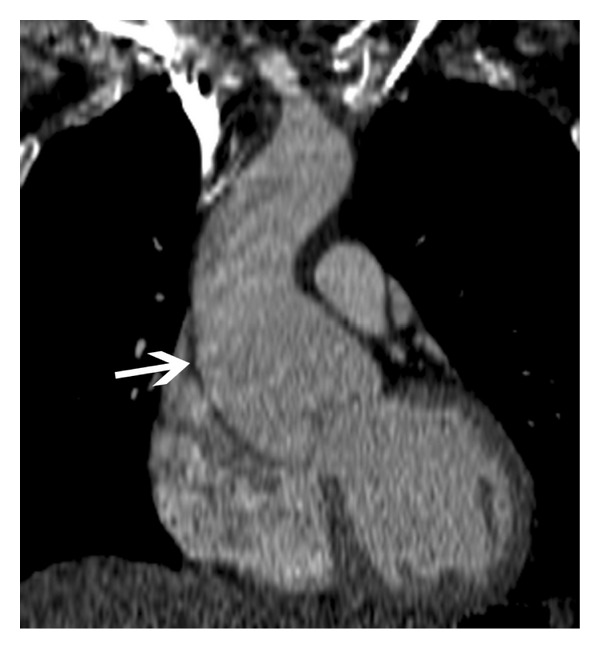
Annuloaortic ectasia—coronal oblique MRI image in a 30-year-old male with Marfan syndrome shows dilated aortic root and ascending aorta with effacement of the sinotubular junction (arrow) consistent with annuloaortic ectasia.
